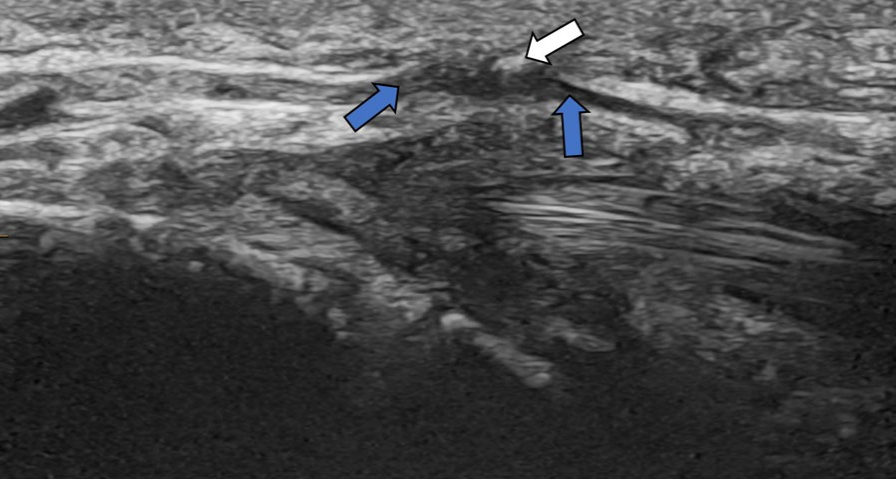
Ultrasound thumb in patient with right thumb pain post trigger finger release. There is fusiform enlargement of the digital nerve (blue arrows) palmar-radial to the site of the prior A1 pulley release, extending over a length of 5 mm. Sonographic palpation elicited the usual symptom of pain radiating down the thumb. There is an echogenic focus (white arrow) immediately superficial to the nerve which may represent suture material (or calcification). This is consistent with entrapment given the proximity to the site of prior intervention
